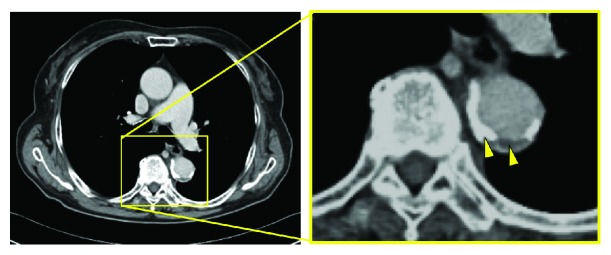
CT showing thrombosed aortic dissection in the thoracic region.
